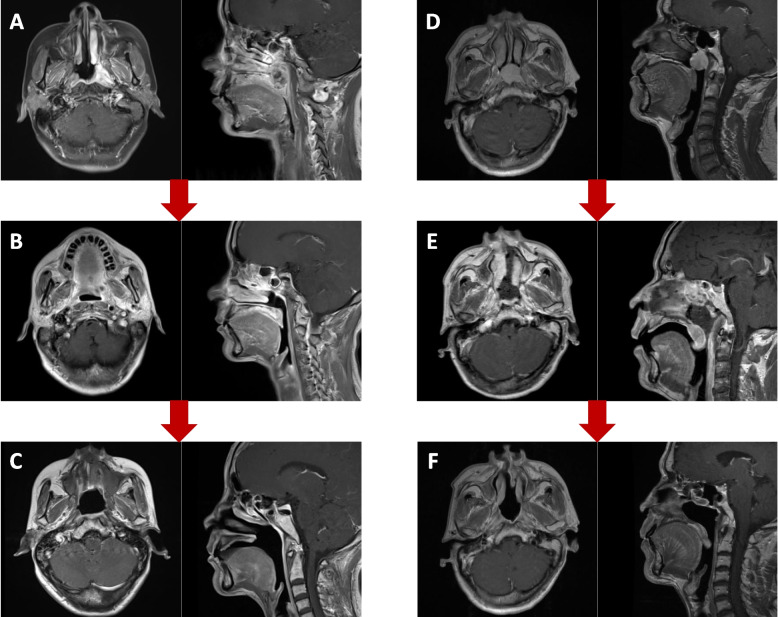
Response to therapy and survival outcomes. Gadolinium–enhanced magnetic resonance images taken (A) at the time of NACC before primary RT, (B) of residual tumour after primary RT, (C) at 6 months of subsequent surgery, (D) at the time of NACC before primary surgery, (E) at 1 week of primary surgery, and (F) at 6 months of postoperative RT. RT, radiotherapy; NACC, nasopharyngeal adenoid cystic carcinoma
